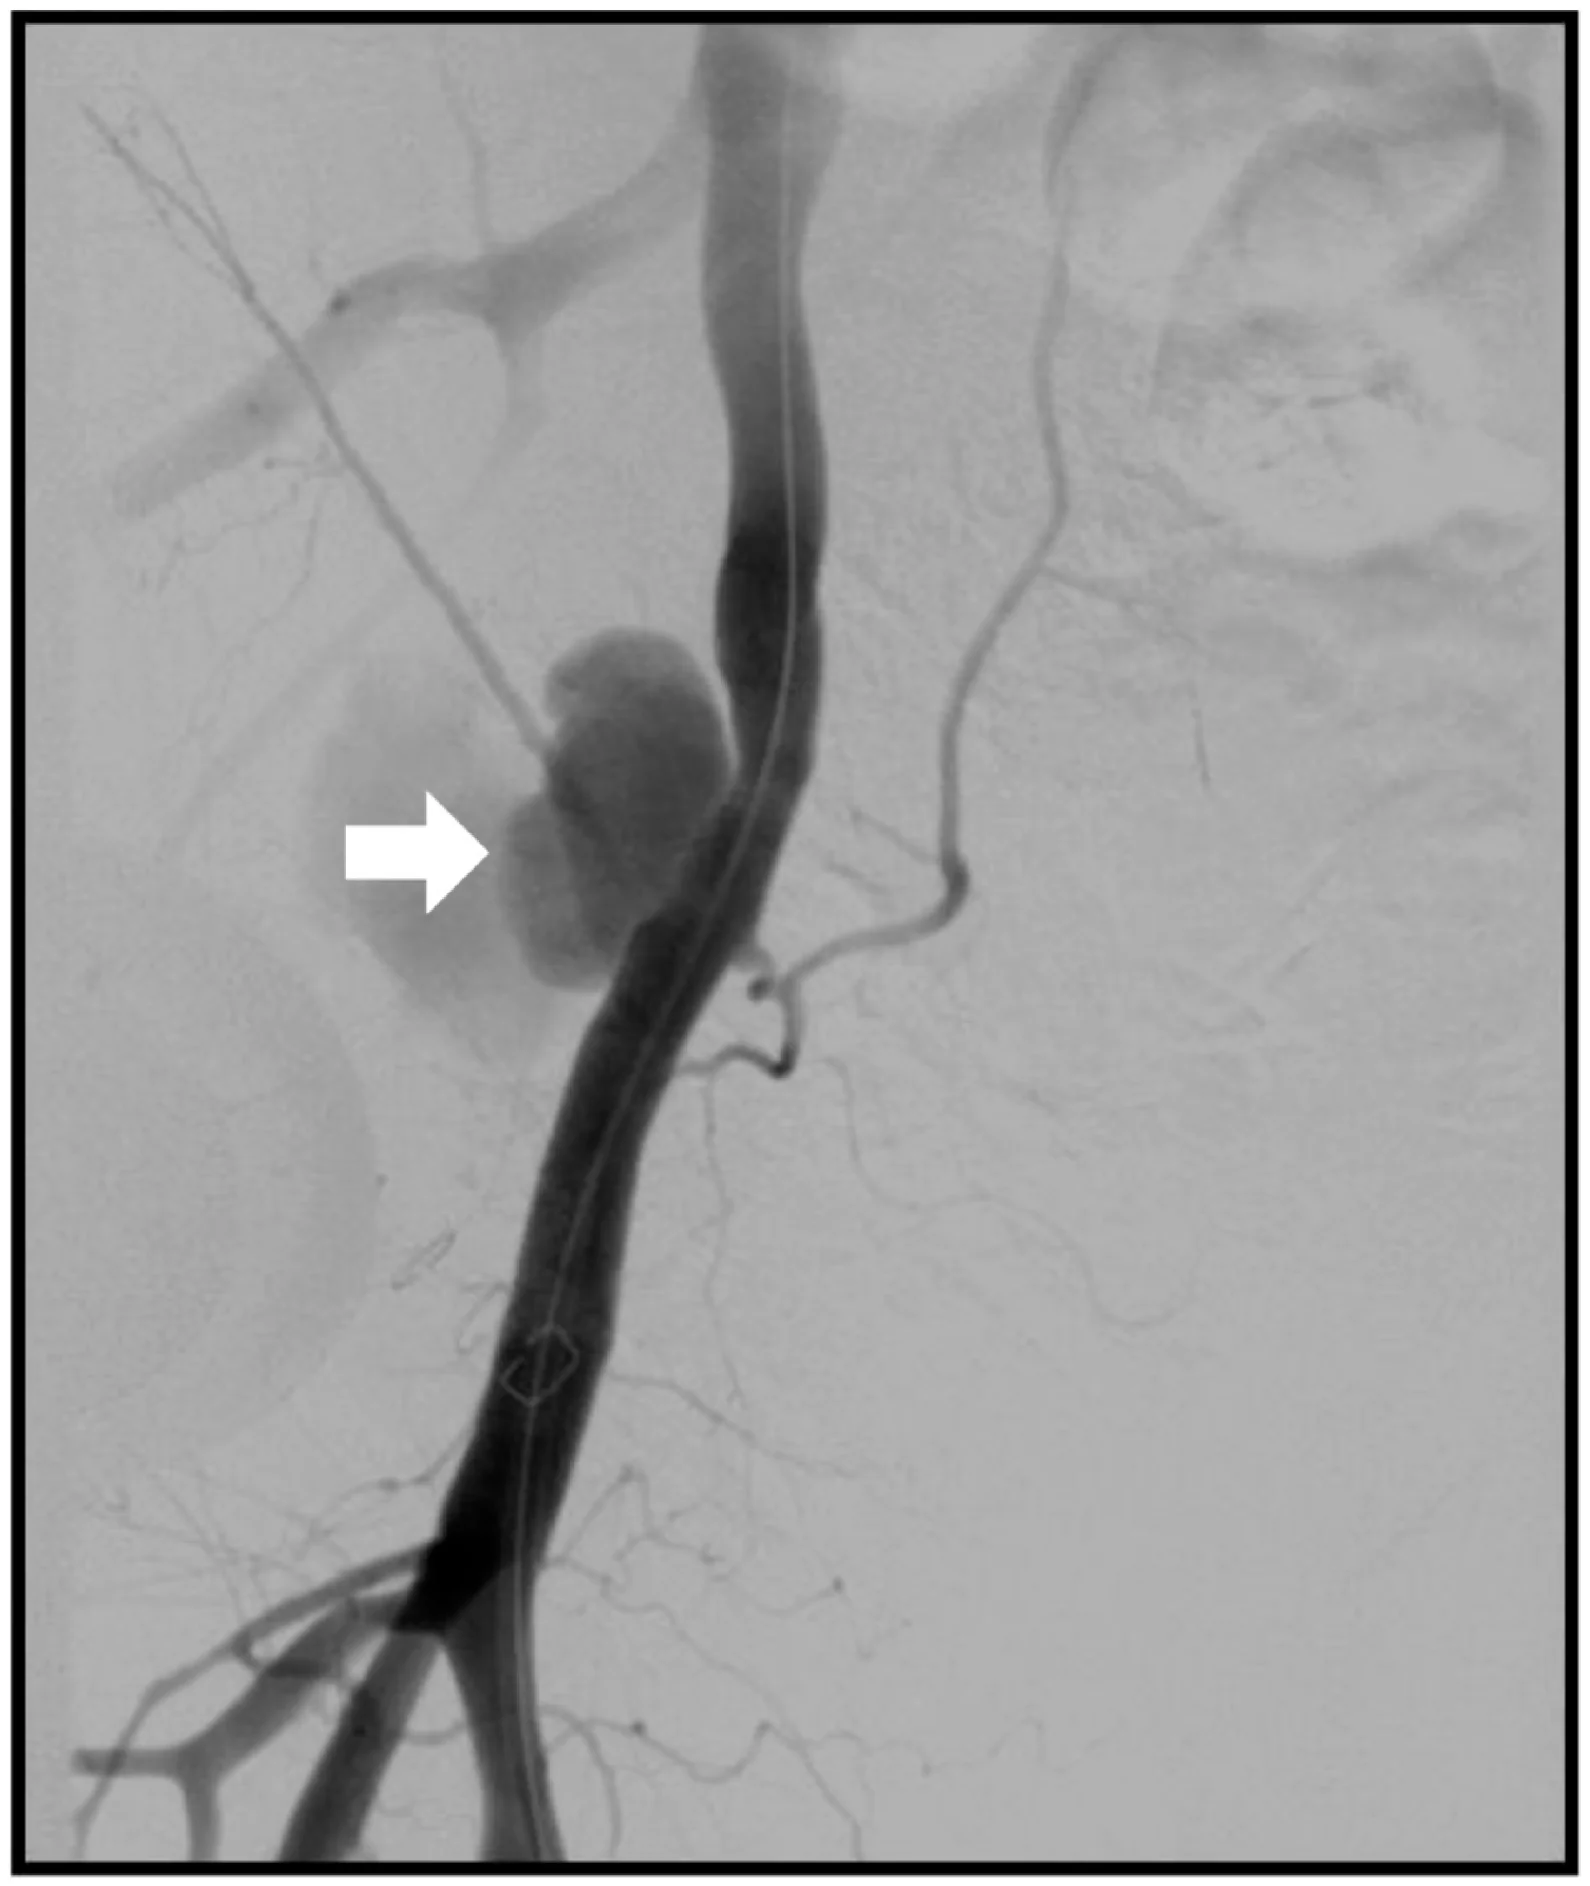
Digital subtraction angiography before stent–graft deployment confirms active extravasation from the right external iliac artery at the level of the inguinal ligament (arrow), corresponding to the bleeding site identified on computed tomography angiography (CTA).
